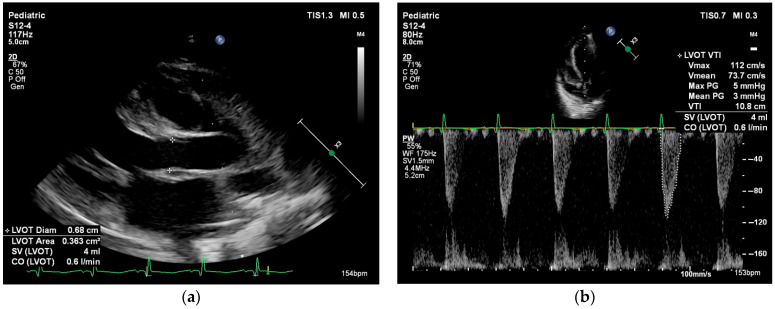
Cardiac output measurement performed in the parasternal long axis view in a neonate with myocarditis. Cardiac output = stroke volume × heart rate. Stroke volume = velocity time integral × cross-sectional area. (a) Left ventricle outflow tract (LVOT) cross-sectional area = π × (LVOT diameter/2)^2^. (b) Velocity time integral is measured using pulsed wave Doppler at the level of the LVOT.
